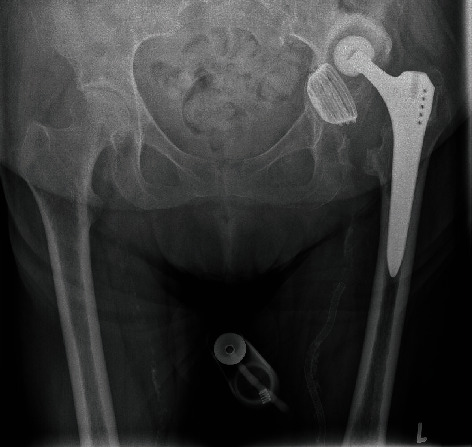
The pelvic radiographs demonstrate dislocation of left total hip replacement, protrusio acetabuli, formation of pseudoarthrosis, and gross loosening of the screw cup. No signs of loosening or fracture of the Zweymüller type femoral stem. Incidental femoral stent on the left.
